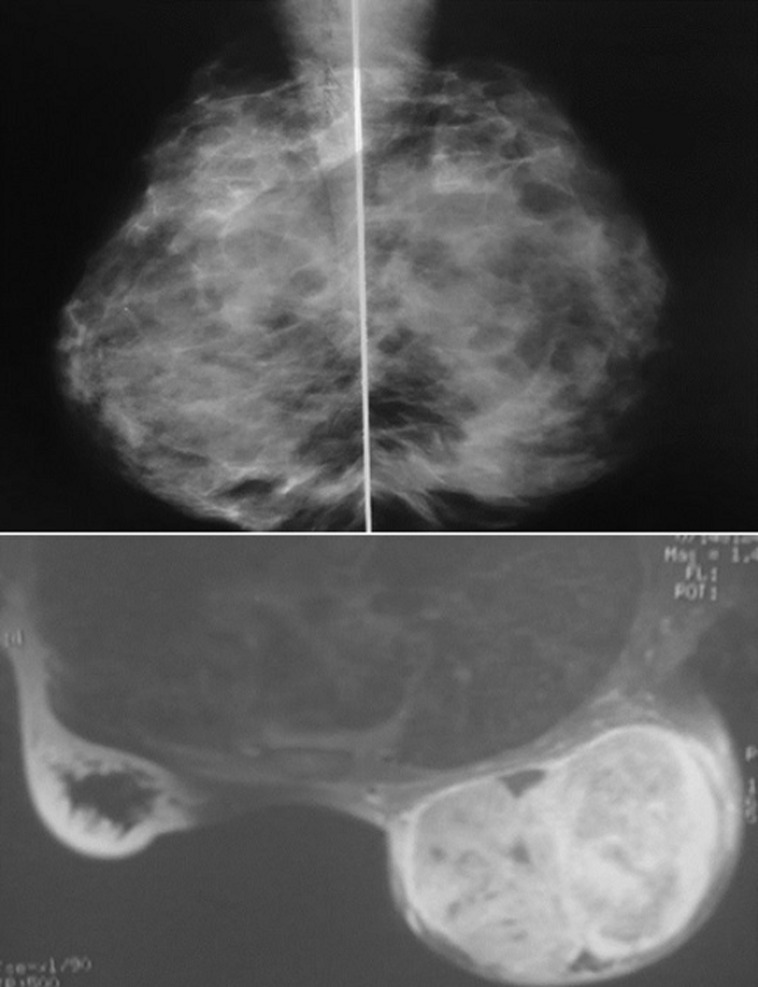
mammogram of the left breast, with transparent, fairly dense X-ray appearance; MRI appearance of a voluminous well-limited echogenic formation, with posterior reinforcement of the right breast of 127 x 110 x 103 mm
